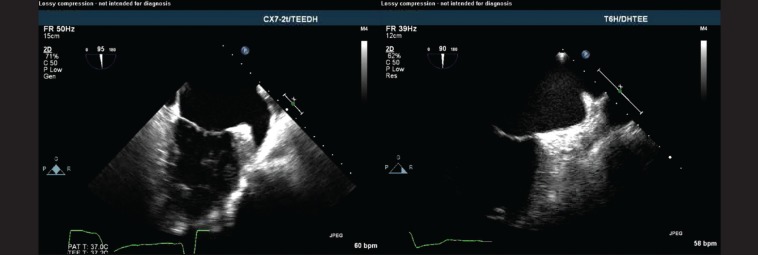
Transesophageal echocardiogram, showing the complete exclusion of the left atrial appendage (LAA). A) Mid esophageal two-chamber view, showing the LAA (arrow) before exclusion. B) Mid esophageal two-chamber view, showing the smooth wall of the left atrium (arrow) immediately after the LAA exclusion with the LARIAT snare
